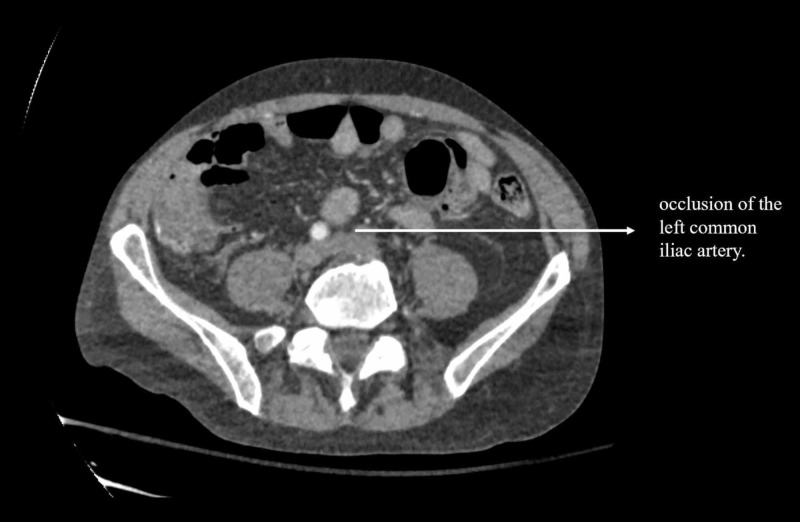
CT of the abdomen and pelvis with contrast demonstrating occlusion of the left common iliac artery.
